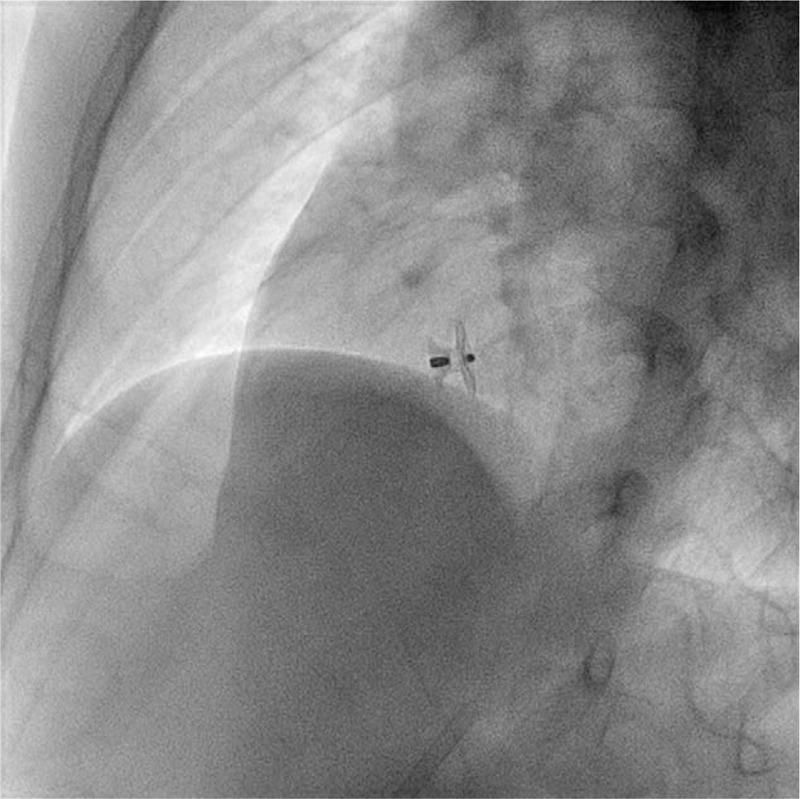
Chest X-ray showed the device location.
